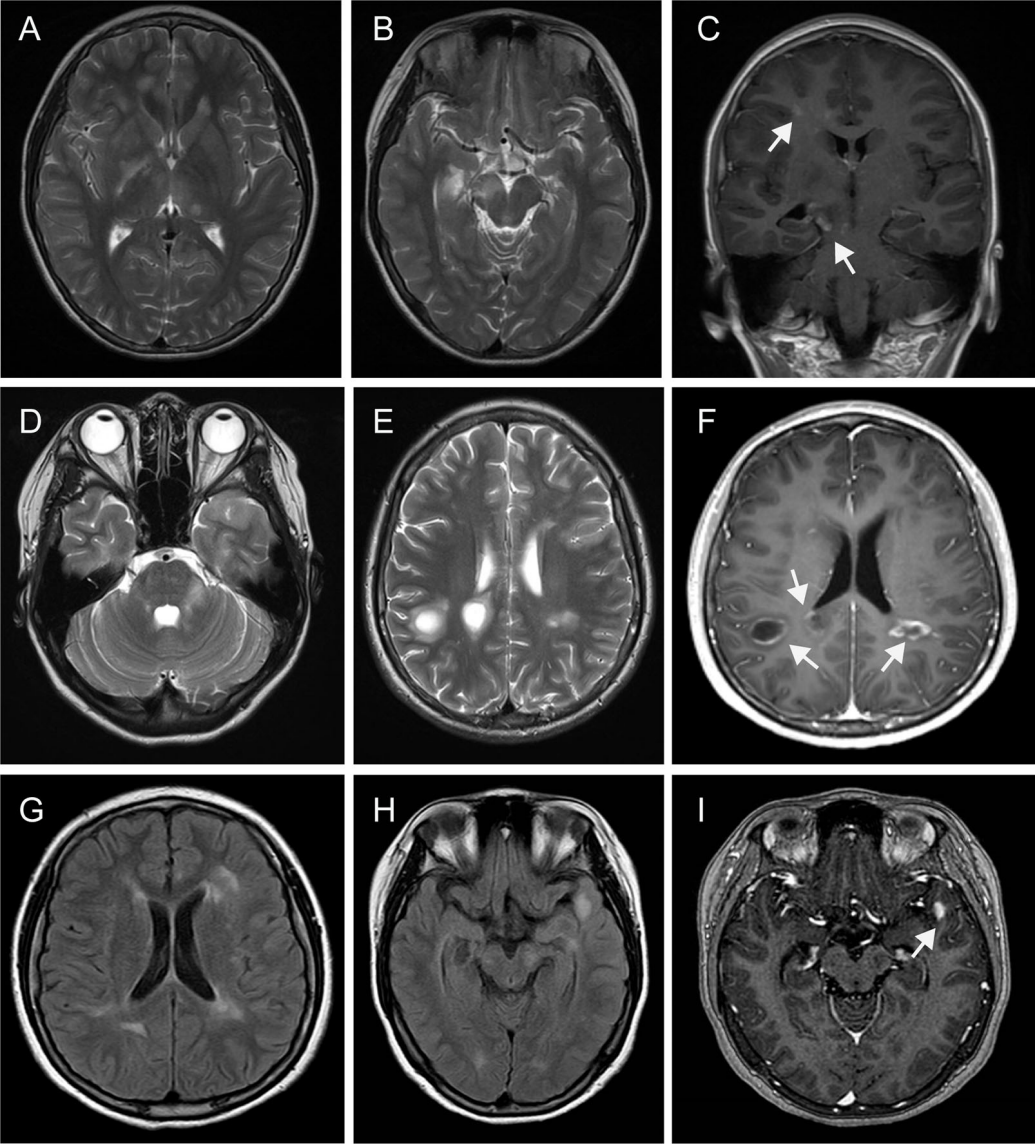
Magnetic resonance imaging findings. MRI performed in July 2012 demonstrated multiple T2 hyperintense lesions (periventricular, juxtacortical, thalamus, basal ganglia, caudex cerebri) (A, B), some of them obtained with gadolinium enhancement [(C), arrowhead]. Repeated MRI in Jan 2020 revealed multiple T2 hyperintense lesions (juxtacortical, periventricular, left brachium pontis), with some lesions appearing as “fried egg sign” (D, E). Some of them with enhancement lesions appeared as “open sing sign” [(F), arrowhead]. MRI in May 2020 exhibited multiple FLAIR hyperintense lesions (periventricular, juxtacortical, left caudex cerebri, left temporal lobe) (G, H), with a left temporal lobe lesion showing gadolinium enhancement [(I), arrowhead]. MRI, Magnetic resonance imaging; FLAIR, fluid attenuated inversion recovery.
